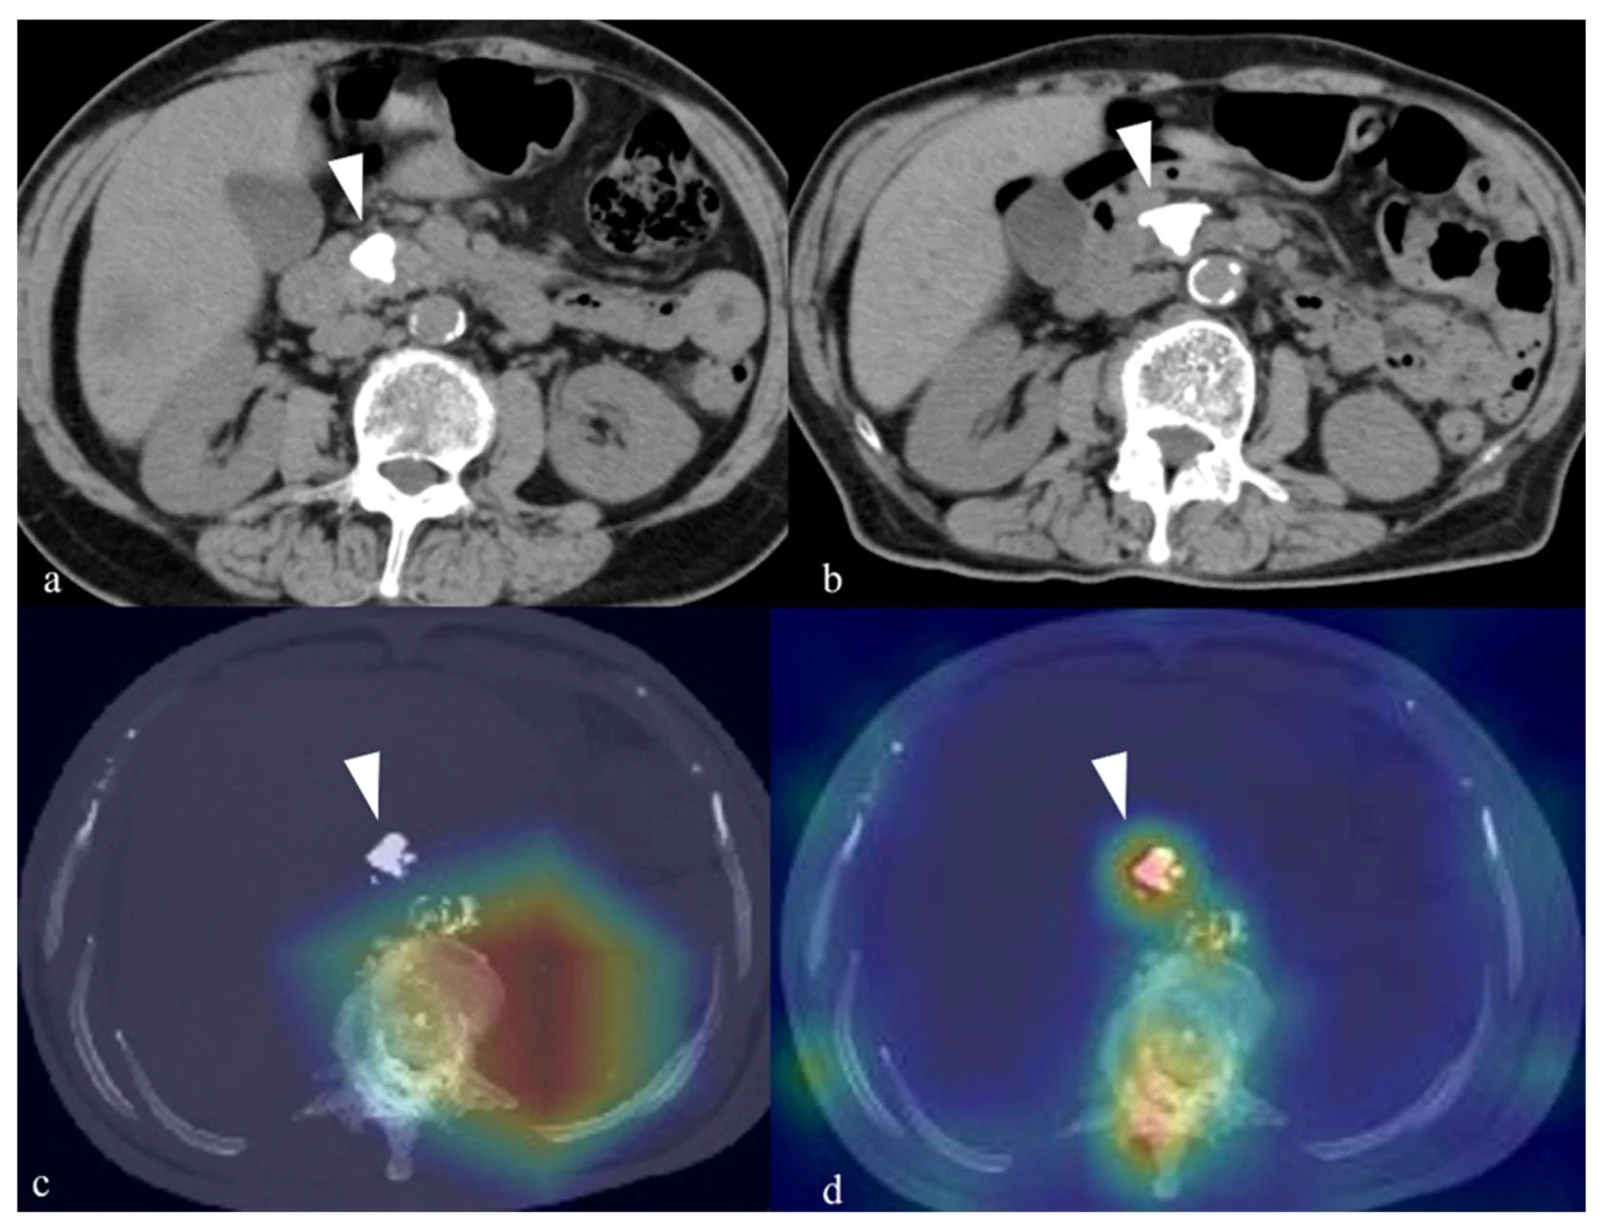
A case of unsuccessful pancreatic stone clearance following nonsurgical treatment. (a) Pretreatment CT image of a patient with asymptomatic idiopathic chronic pancreatitis. A 20-mm pancreatic stone is present in the main pancreatic duct within the pancreatic head (arrowhead). (b) Post-treatment CT image. After a total of 19 ESWL sessions, the stone in the pancreatic head remained (arrowhead), representing unsuccessful stone clearance. ResNet50 predicted unsuccessful pancreatic stone clearance with a probability of 62%, while the ViT-L/16 predicted unsuccessful clearance with a probability of 61%. (c) Grad-CAM visualization of a ResNet50. The highlighted region does not correspond to the pancreatic stone (arrowhead), indicating that the model attended to a different region during prediction. (d) Attention Map visualization of the ViT-L/16. The highlighted region corresponds appropriately to the pancreatic stone (arrowhead), indicating that the model attended to the anatomically relevant region.
